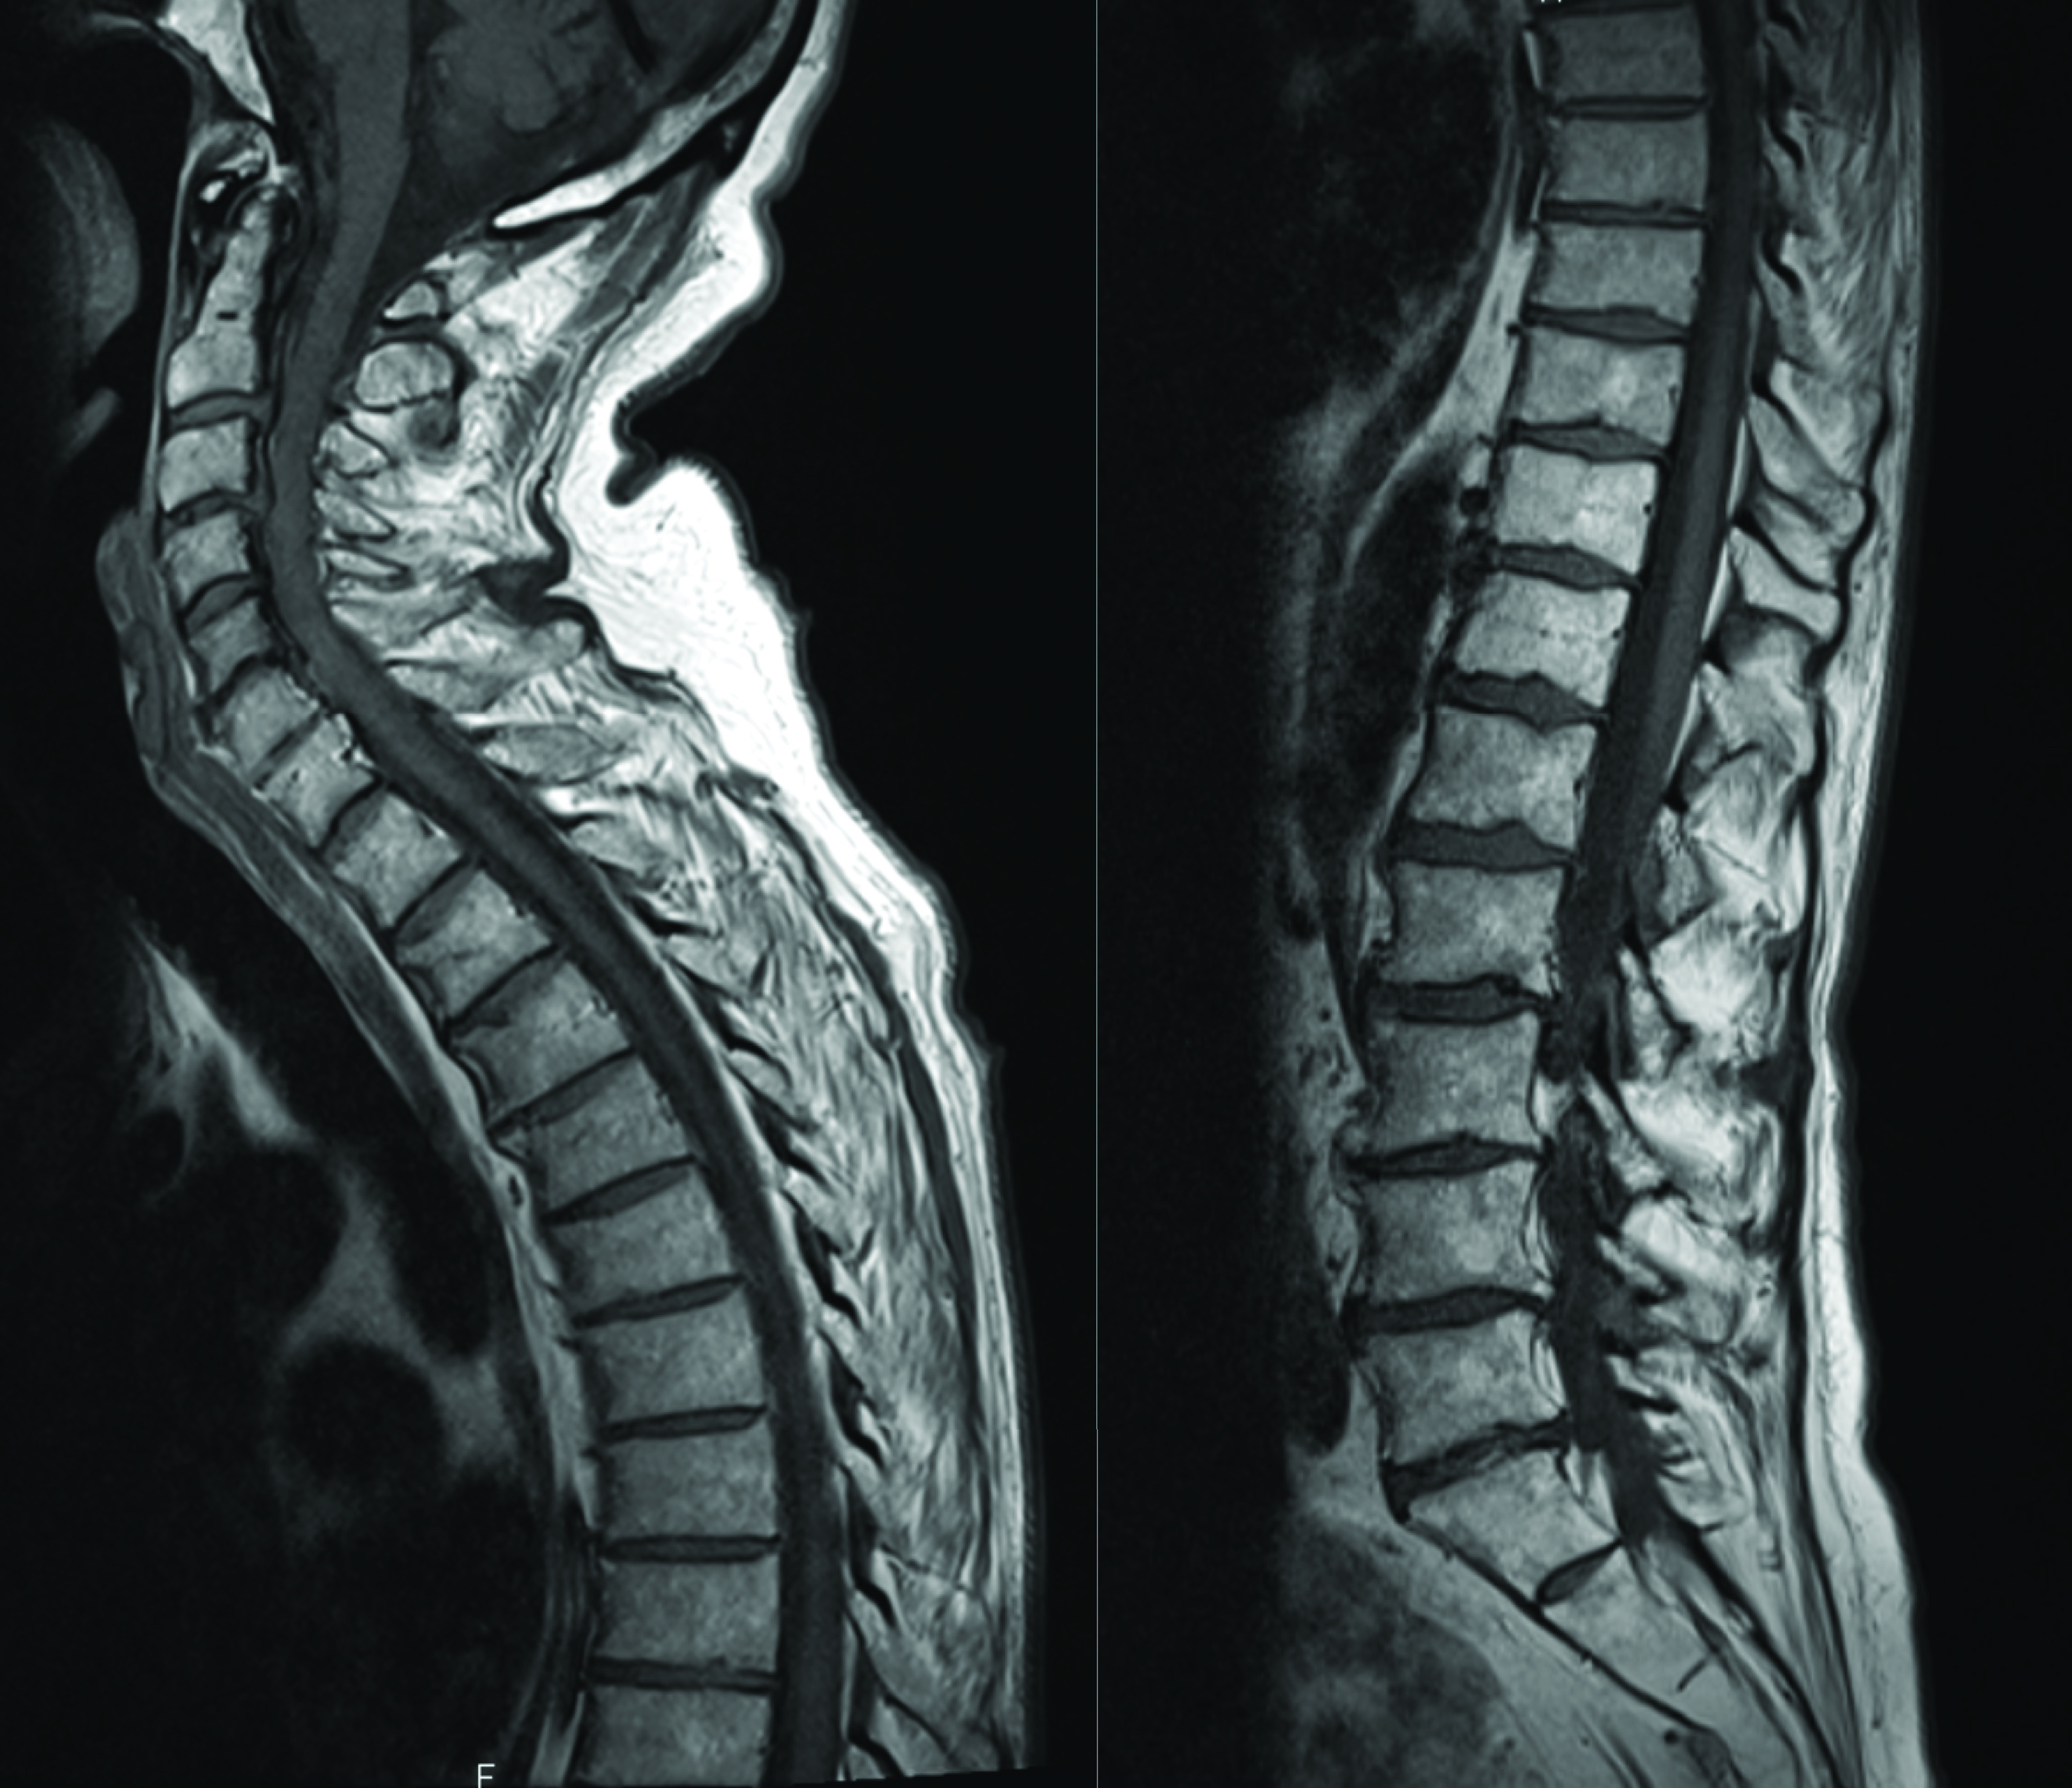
Axial skeletal MRI at cancer diagnosis showing no bone lesion.
